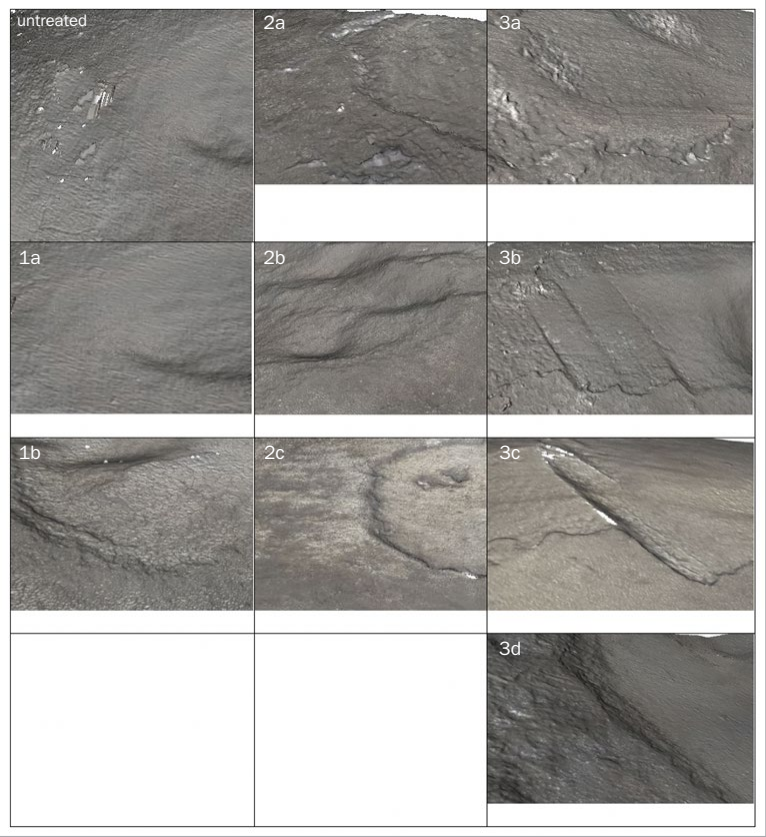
Cementum at 20X magnification: (1a) air polishing only or (1b) with rubber-cup polishing; (2a) ultrasonic scaling only or (2b) with air polishing or (2c) with rubber-cup polishing; (3a) hand scaling only or (3b) with air polishing or (3c) with rubber-cup polishing or (3d) with both.
